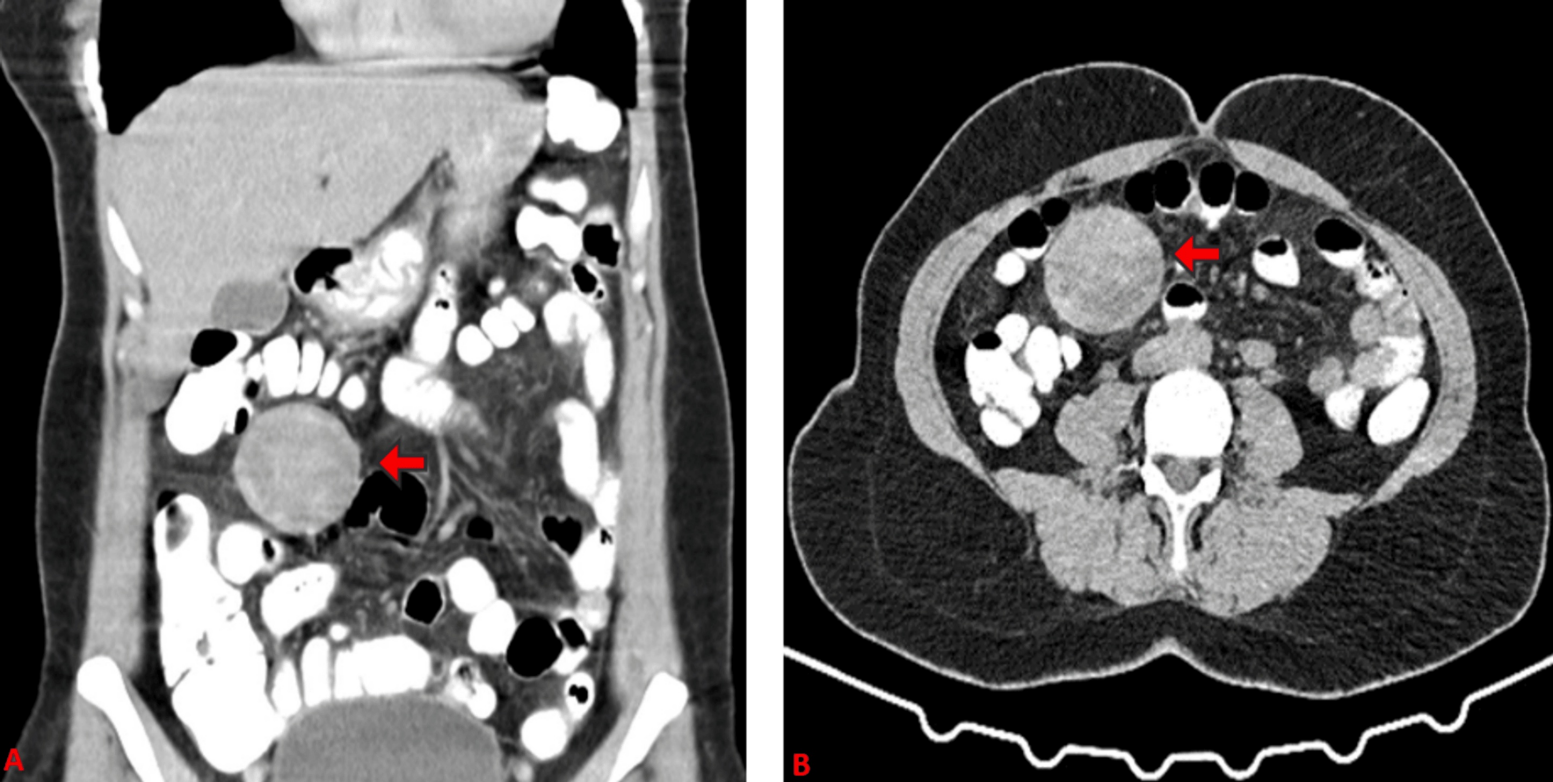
CT of the abdomen coronal view (A) and axial view (B) indicating a spherical mass (5.4 x 5 cm) with a thick enhanced wall after intravenous contrast (red arrows). The mass is located in the larger omentum in close relation with the jejunum with no sign of obstruction or intestinal ischemia.
